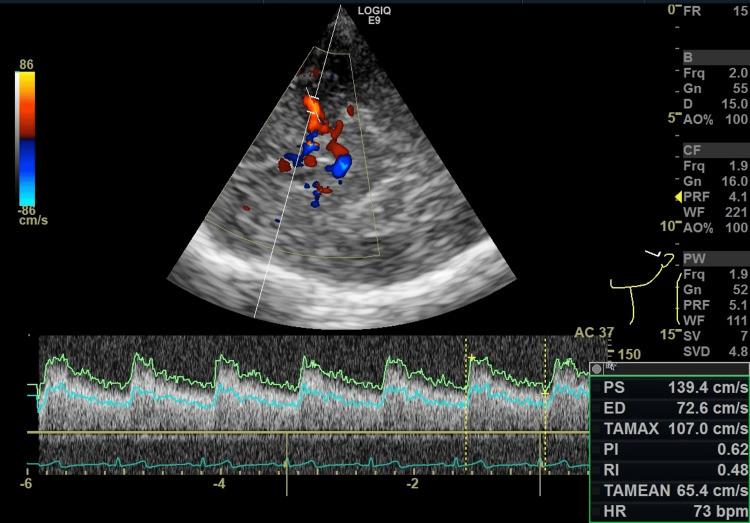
Representative TCCFI measurement of the MCA Color Doppler imaging identifies the MCA through the temporal bone window, and pulsed-wave Doppler is used to record the flow velocity waveform. The angle of insonation (AC = 37° in this example) is adjusted along the vessel course, and the TAMX is automatically calculated over one cardiac cycle by the system software (TAMX = 107 cm/s). TCCFI: Transcranial color flow imaging, TAMX: Time-averaged maximum flow velocity, MCA: Middle cerebral artery, AC: Angle correction
